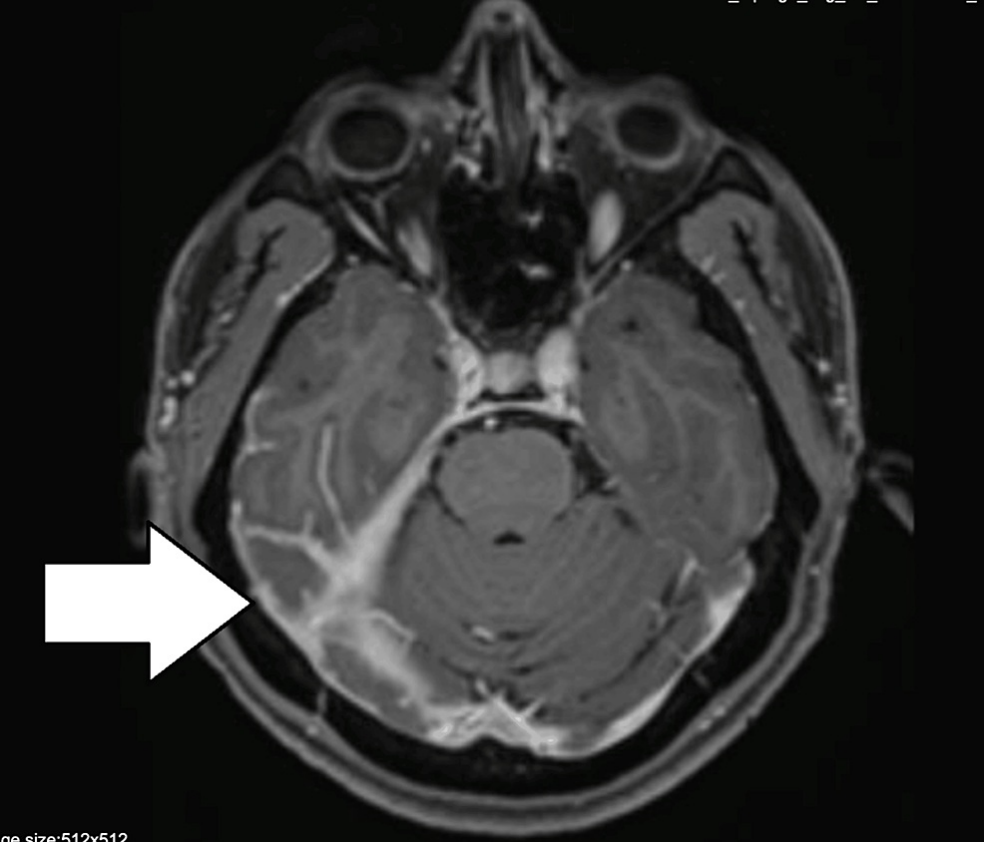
MRI of the brain: T1 axial contrast showing contrast enhancement in the right tentorium cerebelli
